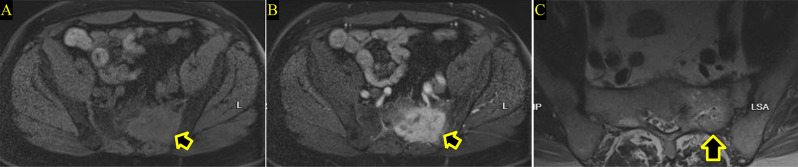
Magnetic resonance imaging (MRI) performed in 2023 with T1 precontrast (A), T1 Post-gadolinium (B) and T2 sequence (C) showing a left sacral mass lesion which exhibit low T1, high T2 signal with marked enhancement in keeping with hypervascular bony lesion (Arrows)
